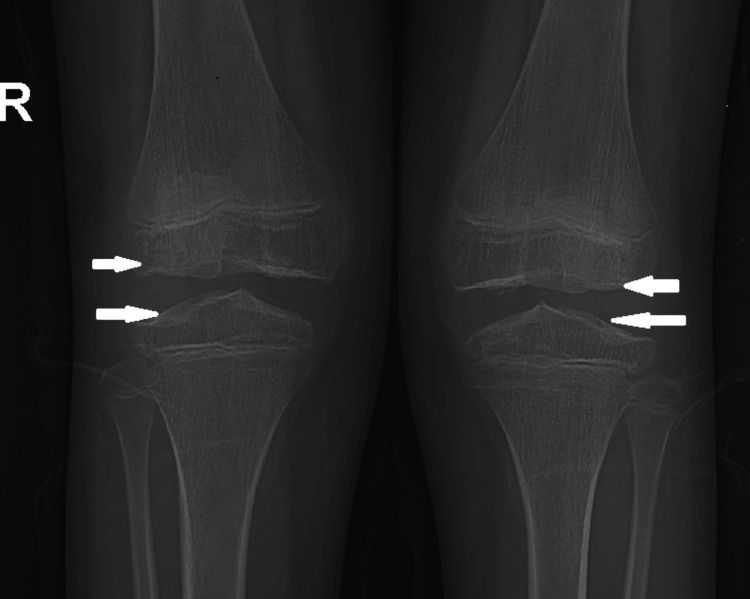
X-ray of both knee joints (AP view) showing irregularities and flattening of epiphyseal ends of femur and tibia
